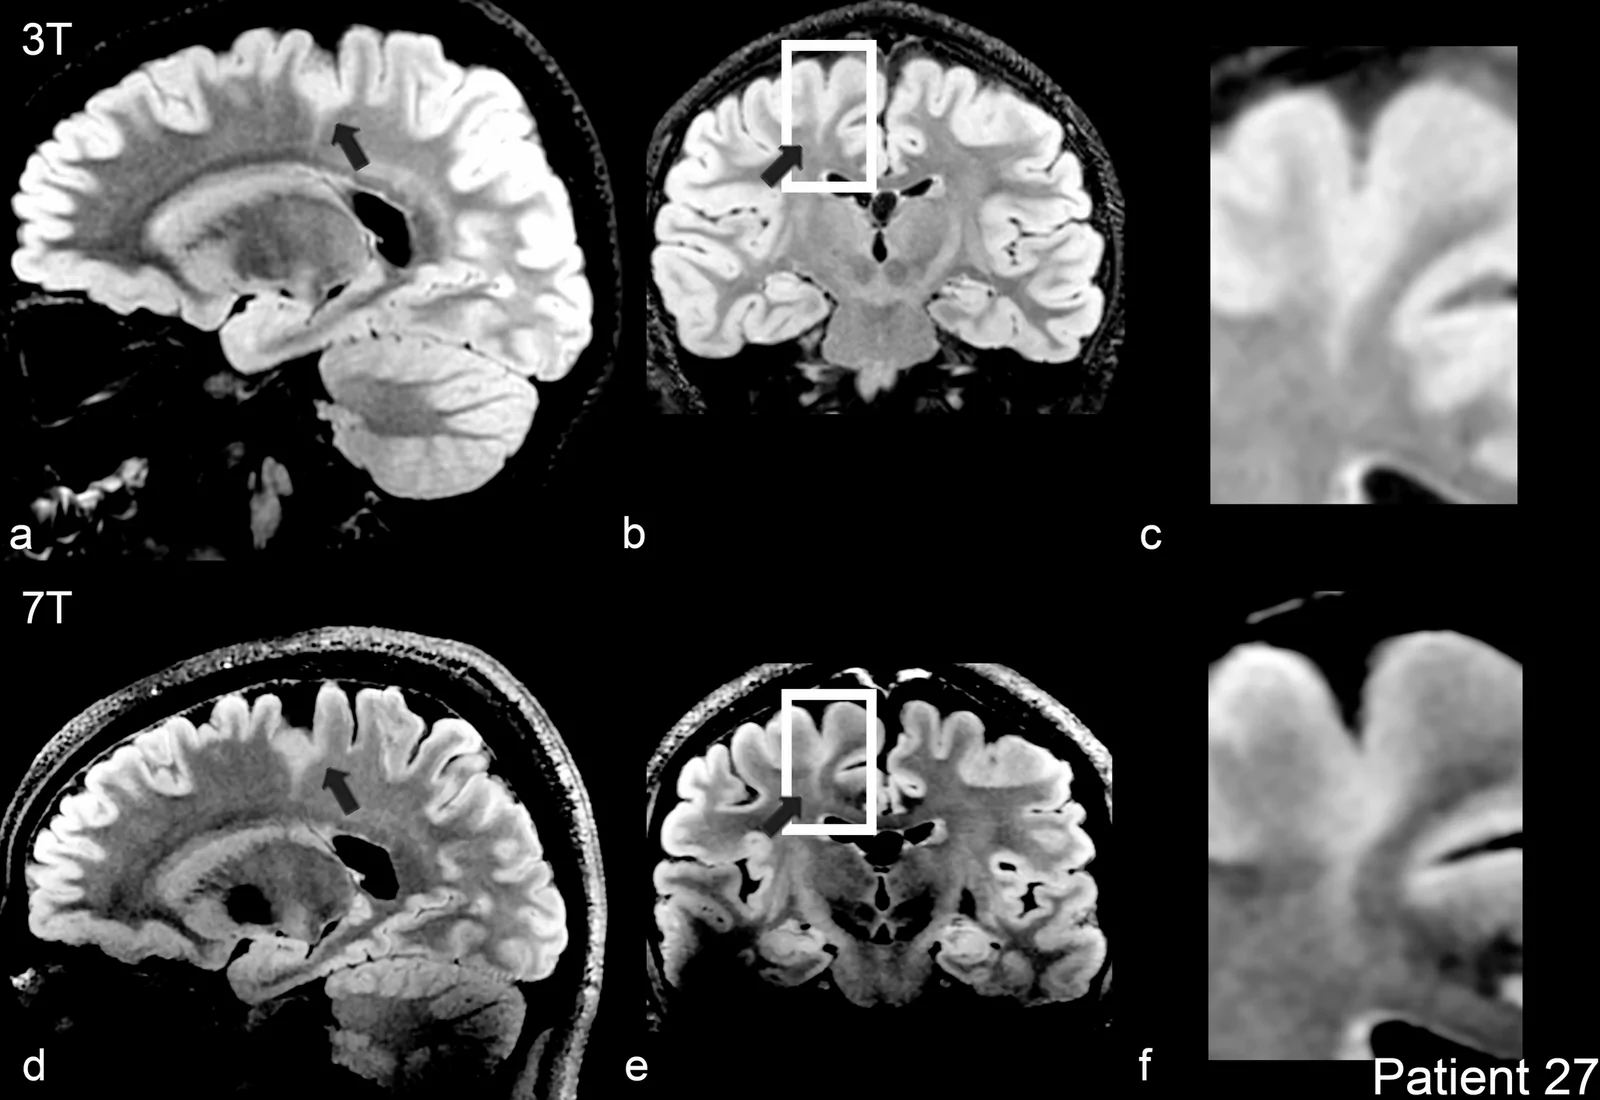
3 T and 7 T MRI in a patient with suspected focal cortical dysplasia (FCD) (Patient 27). Panels a-c show 3 T sagittal (a), coronal (b) and magnified coronal (c) FLAIR images, while d-f show the corresponding 7 T images. The images demonstrate a transmantle sign, suggestive of FCD-II, and magnified views (c and f) show improved delineation of the sulcus with loss of grey matter boundaries at 7 T MRI (f) compared with 3 T MRI (c)
